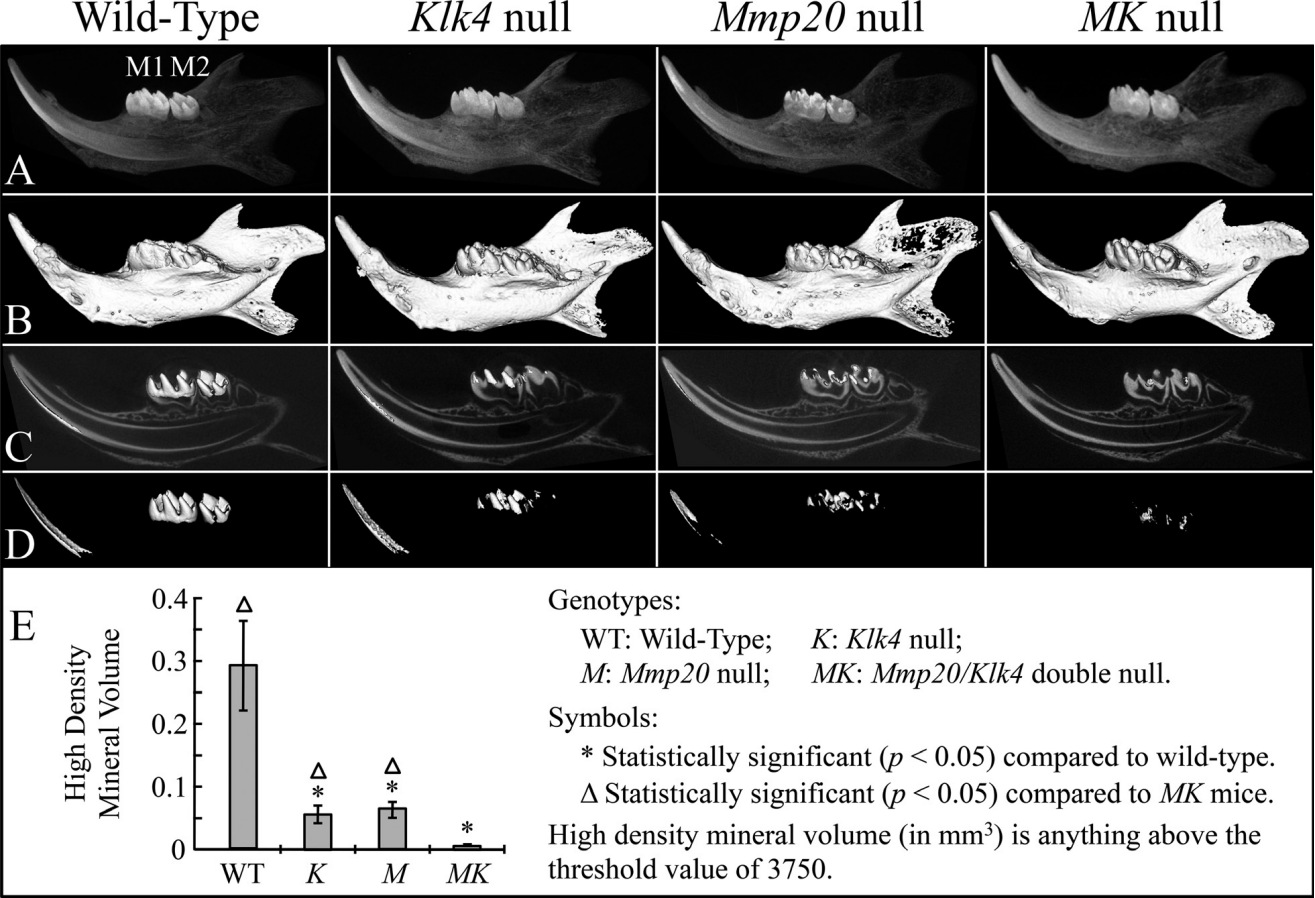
Microcomputed tomography (*μ*
